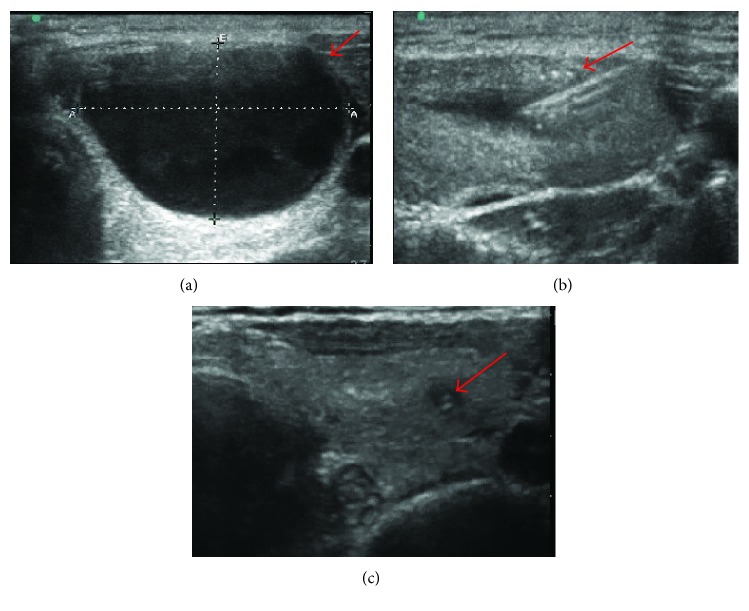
Ultrasonographic images of a 23-year-old woman before, during, and after treatment: (a) a cystic thyroid nodule of ultrasonographic images before PPI treatment; (b) during PPI treatment; (c) 12 months after PPI treatment; the nodule decreased significantly (arrow). PPI: percutaneous polidocanol injection.
